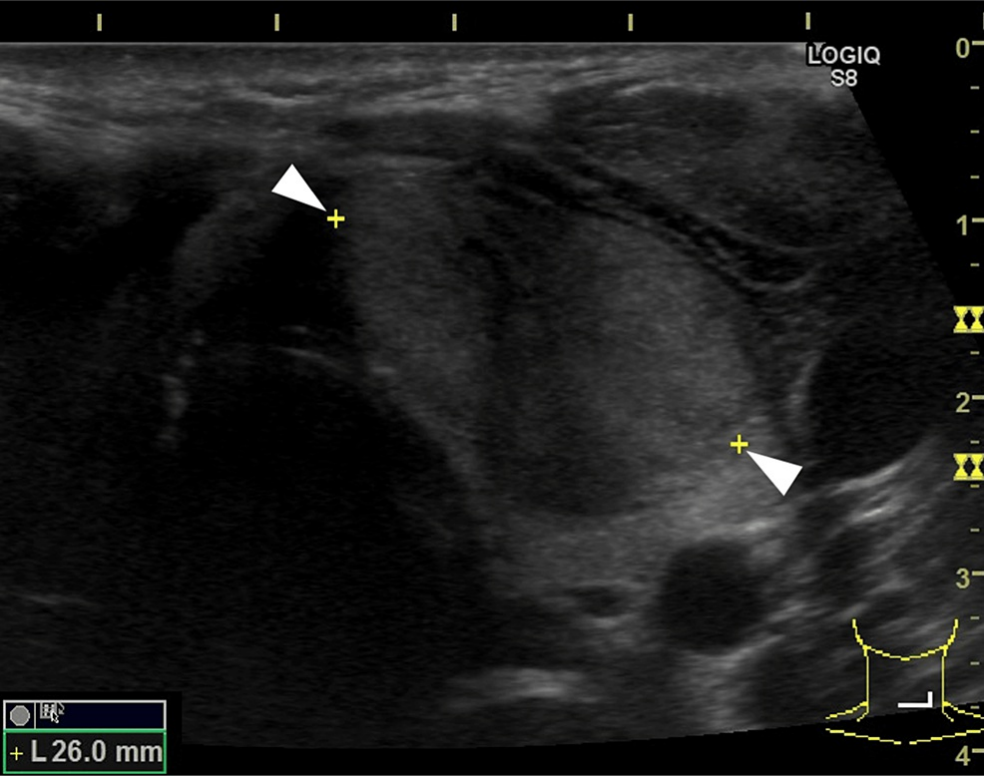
Ultrasound Ultrasound: 26×20×16 mm hypoechoic mass in the left lobe
